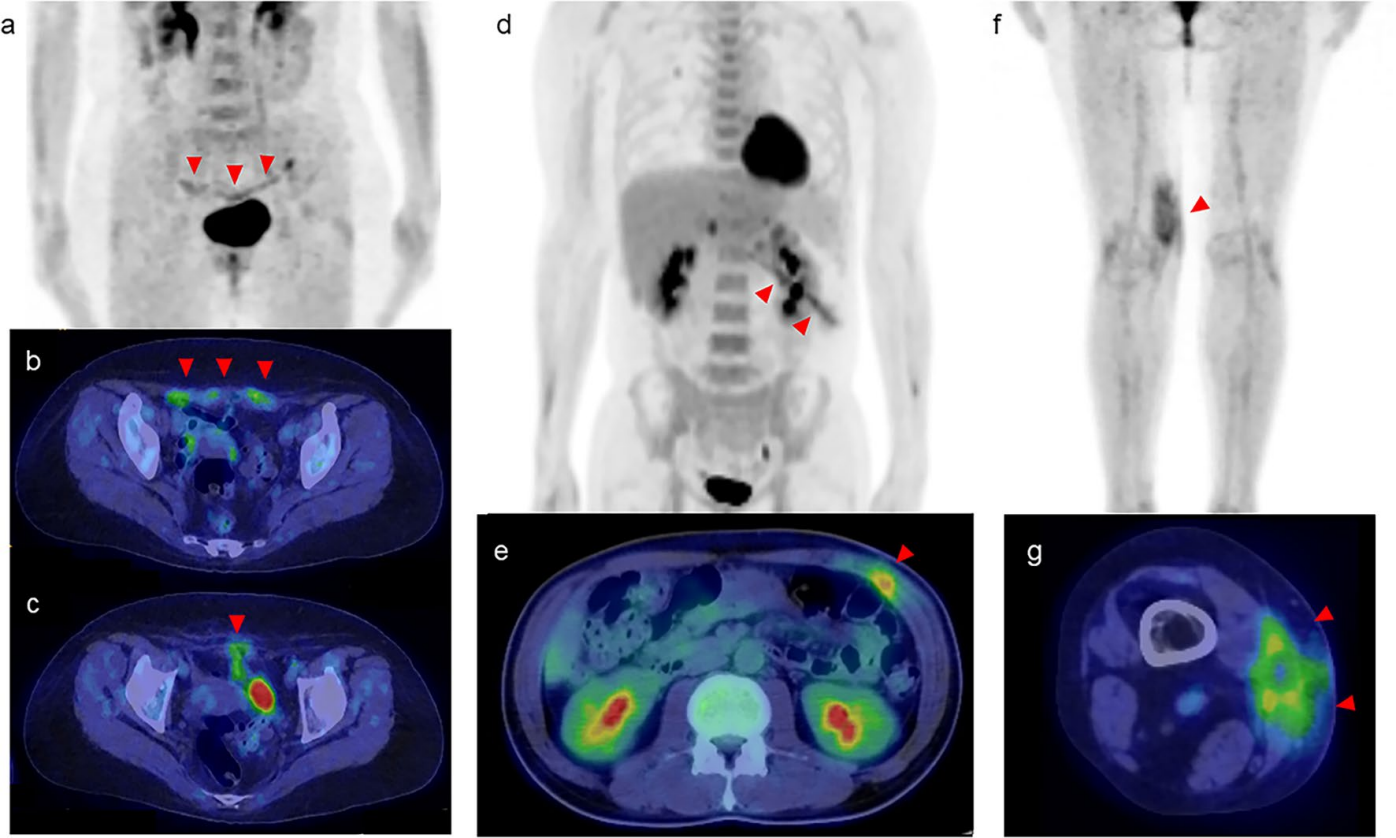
a, d, and f FDG-PET MIP images. b, c, e, and g Axial FDG-PET/CT images. Various degrees of FDG uptake (arrowheads) are seen, consistent with the surgical procedure in a short time since the surgery
